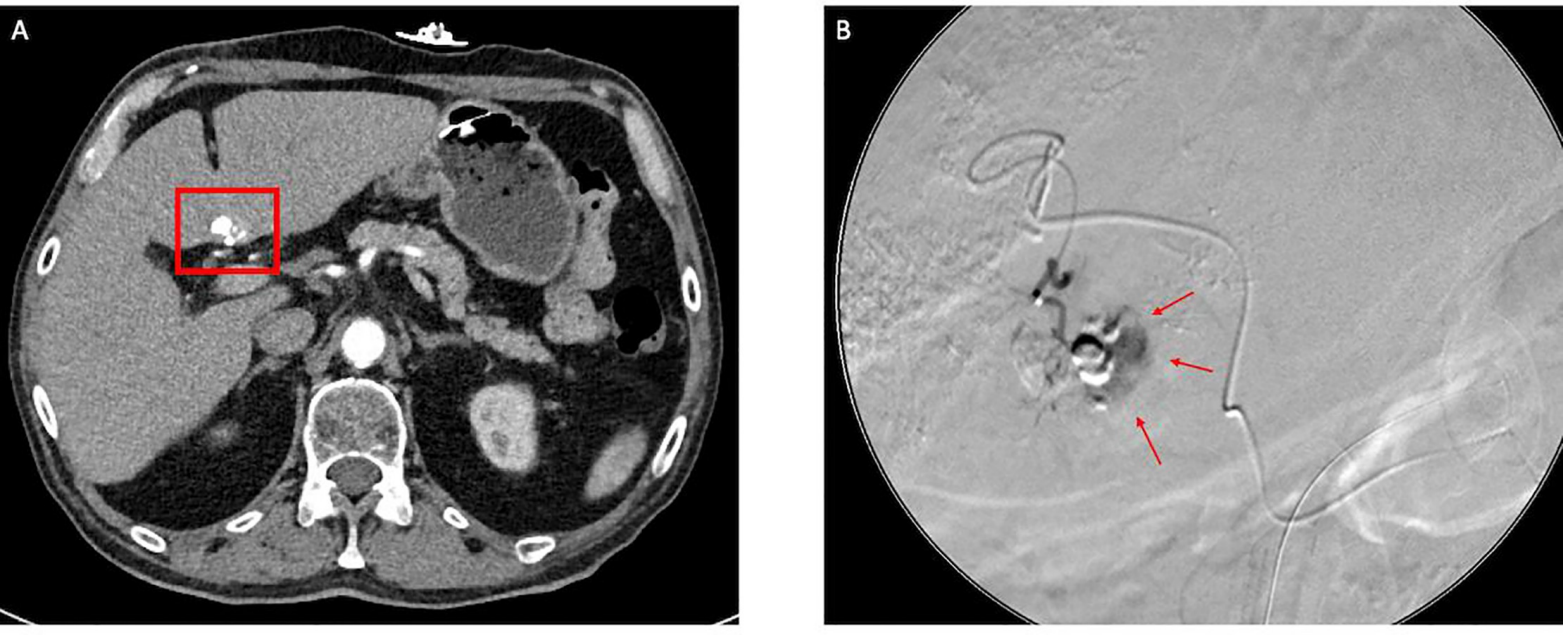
A: Computed tomography in the arterial phase of a patient with a hidden residual tumor; B: DSA of the same patient unveiling the hidden residual tumor. Measuring the density of iodine depositions in this patient, an enhancement score of 17.50 clearly revealed a hidden residual tumor beyond the iodine depositions that was diagnosed afterward in the DSA.
